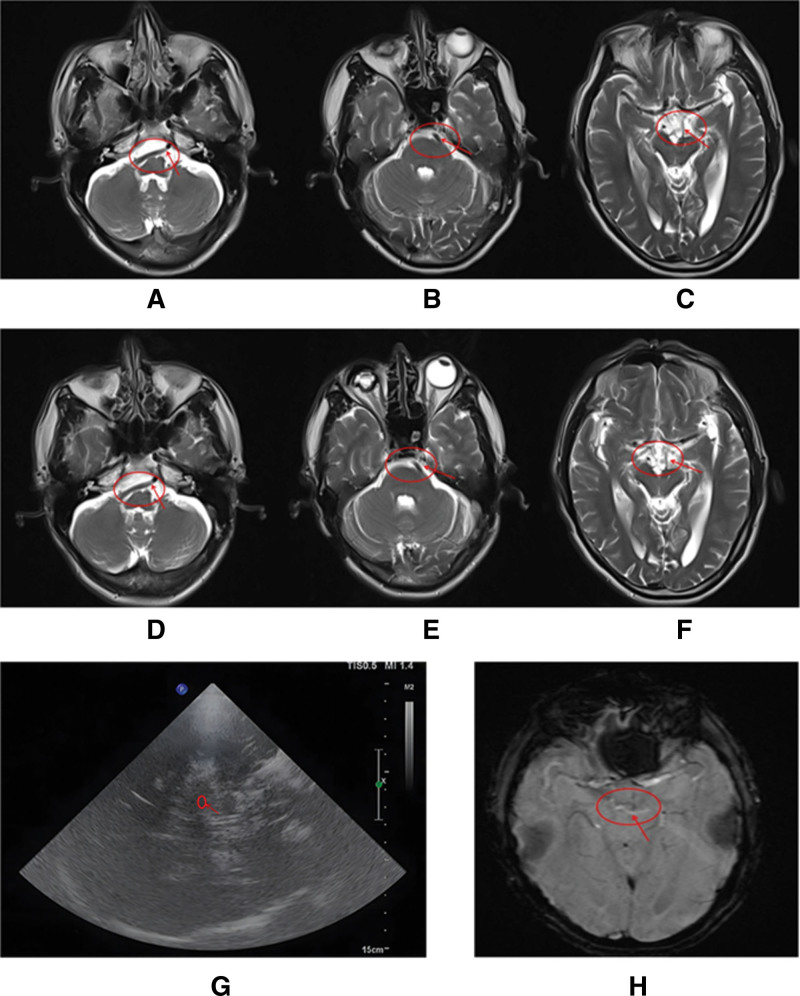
Brain MRI of the patient in 2022 (A–C) and 2024 (D–F) showed a dilation and elongation of basilar artery, which compressed the left midbrain. The severity of tremors was higher in 2024 than in 2022. Cranial ultrasound examination showed strong patchy echoes in substantia nigra (III grade) with an area of >0.2 cm^2^ (G). Susceptibility-weighted imaging also found the dilation and elongation of basilar artery, which compressed both sides of the midbrain (H). Red circles and arrows indicate the lesion sites. MRI = magnetic resonance imaging.
